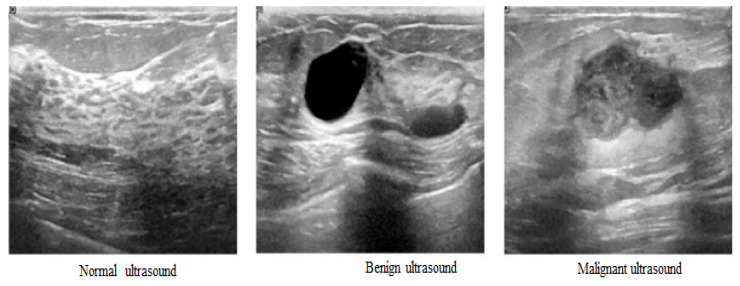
Normal, benign, and malignant ultrasound.
